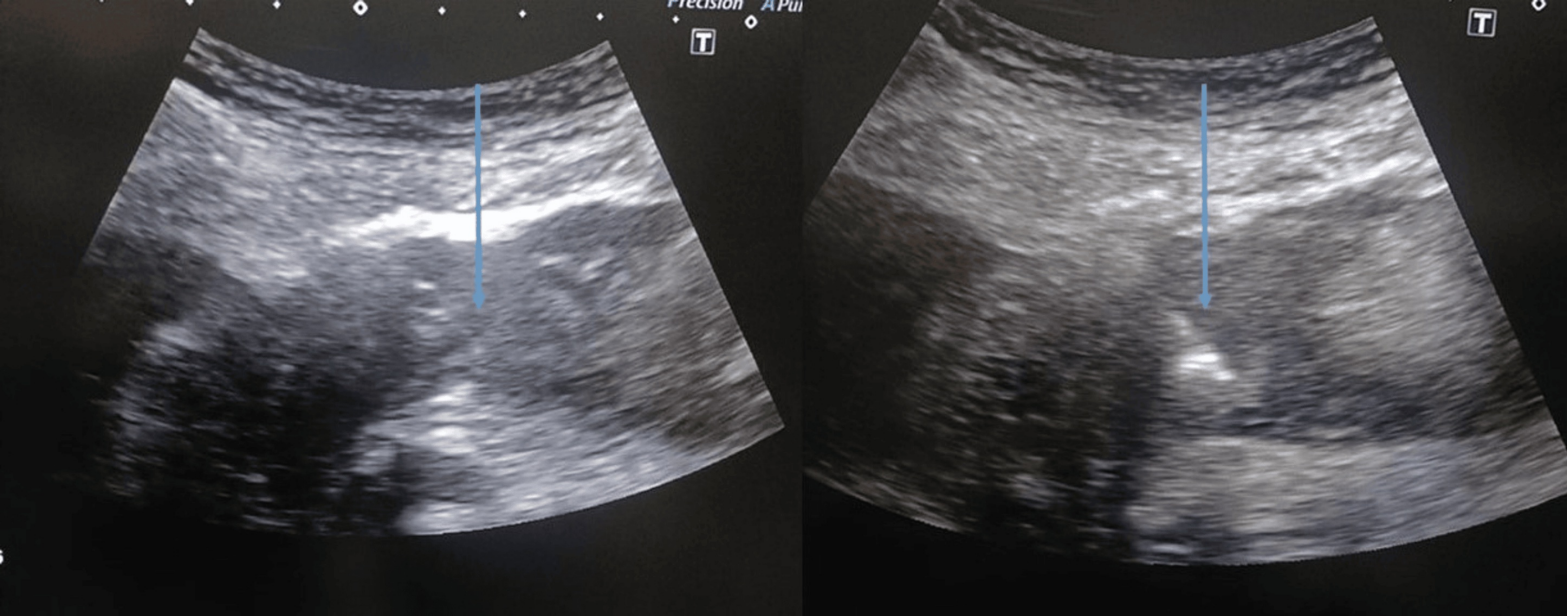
Ultrasound guided needle placement within the thickened omentum (needle tip indicated by blue arrow)
